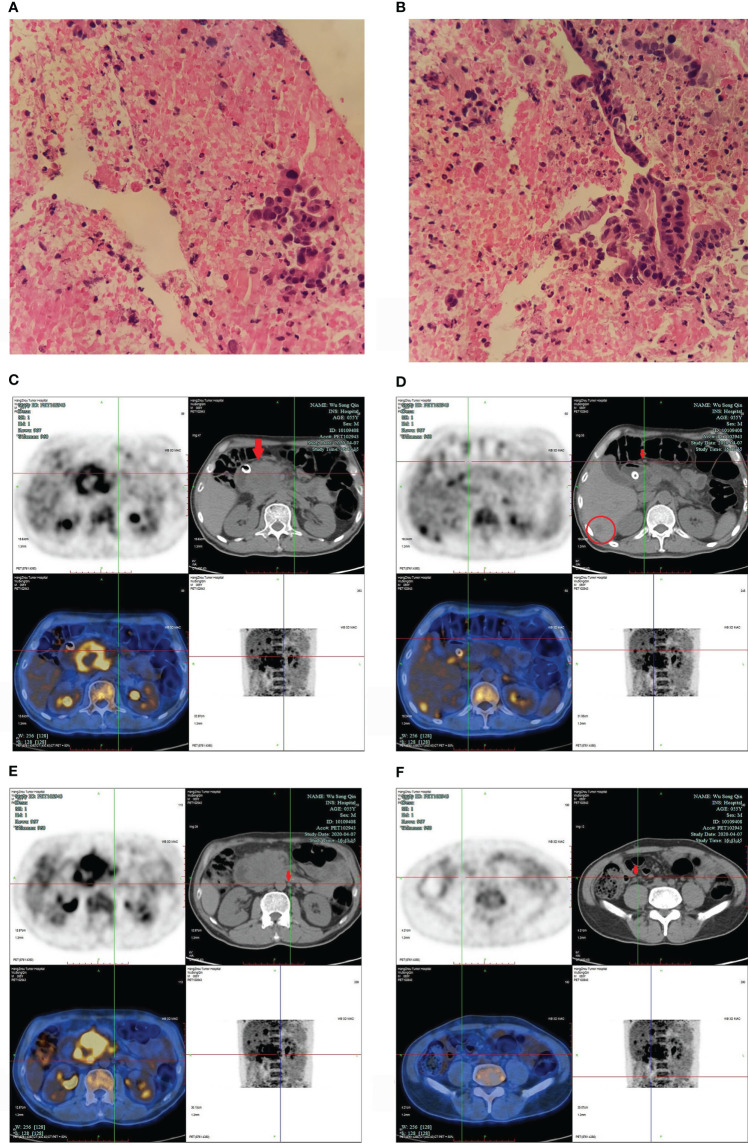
(A, B) pancreatic ductal adenocarcinoma. (A) 200X, (B) 400X. The pancreatic head puncture smear showed significant atypical epithelial mass, irregular nuclei, which was consistent with the changes of adenocarcinoma. (C–F) PET/CT images in April 2020. (C) showed a 6.0cm *6.0 cm mass in pancreatic head (arrow); (D) confirmed enlarged lymph nodes in pancreatic head (arrow), and low-density shadows in liver (within cycle); panels (E, F) revealed enlarged multiple lymph nodes in different sizes in retroperitoneum (arrow).
